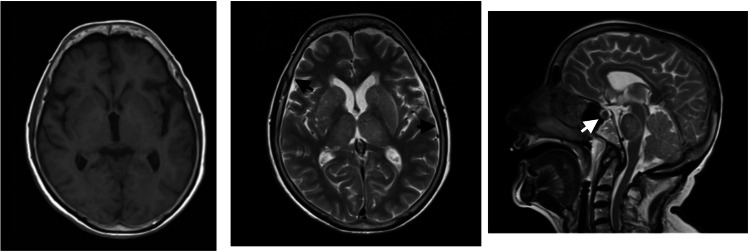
Brain magnetic resonance imaging of patient 2 Extensive edematous changes with a marked predominance of vasogenic edema at the level of the basal ganglia, radiated crowns, and frontal and occipital white matter bilaterally in the pontine region and the cerebellar hemispheres, with the presence of associated cytotoxic edema areas, are observed. Changes consistent with a pseudohypoxic pattern are described in the context of spinal surgical intervention. There was also an engorgement of the pituitary gland (white arrow), diffuse thickening, and pachymeningeal enhancement (black arrows); these findings are compatible with a context of cerebrospinal fluid hypotension.
